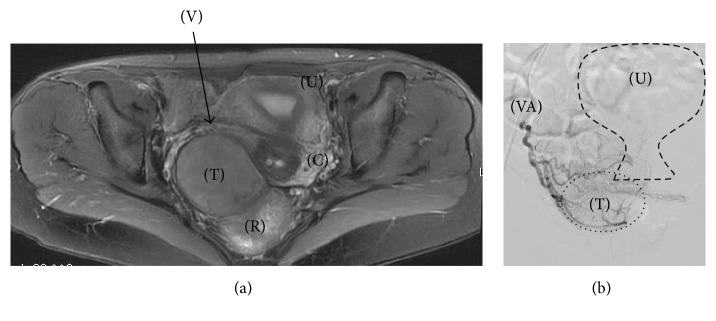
(a) Contrast enhanced MRI radiological analysis. A transverse section shows the tumor (T) anteriorly compressing the uterus corpus (U), cervix (C), and vaginal canal (V), posteriorly compressing the rectum (R). (b) Angiography frontal section image showing that the tumor (T) was nourished from the vaginal artery (VA) and the uterus corpus (U).
